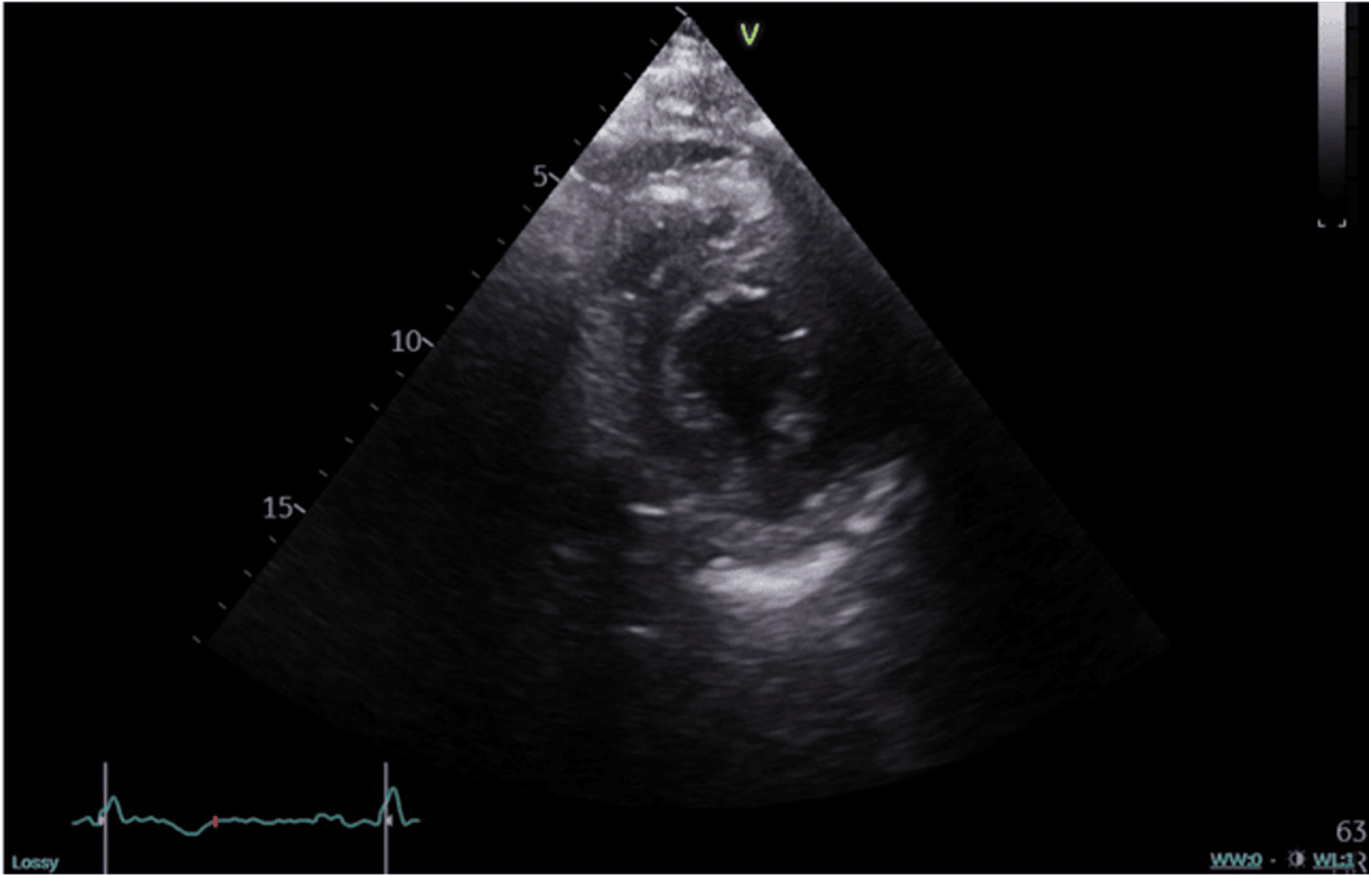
Parasternal short axis view from echocardiogram In this parasternal short axis view from echocardiogram, the cardiac structures are normal. The cardiac mass is not seen.
